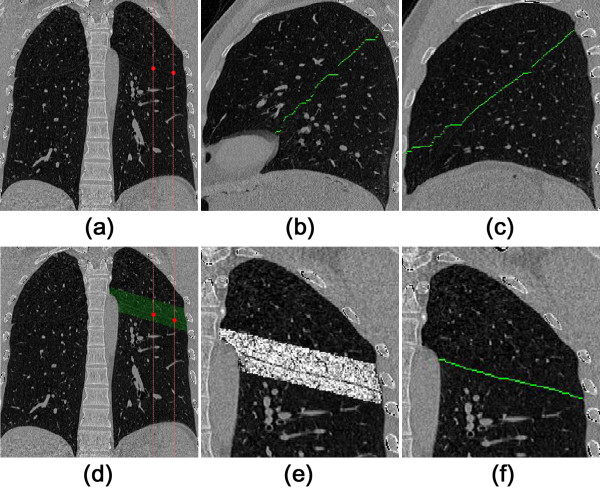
Improved adaptive fissure scanning procedures. (a) coronal CT image; (b) the first sagittal image slice; (c) the second sagittal image slice; (d) coronal CT image with *FR* super imposed; (e) after Hessian matrix enhancement; (f) the final fissure after UCS.
